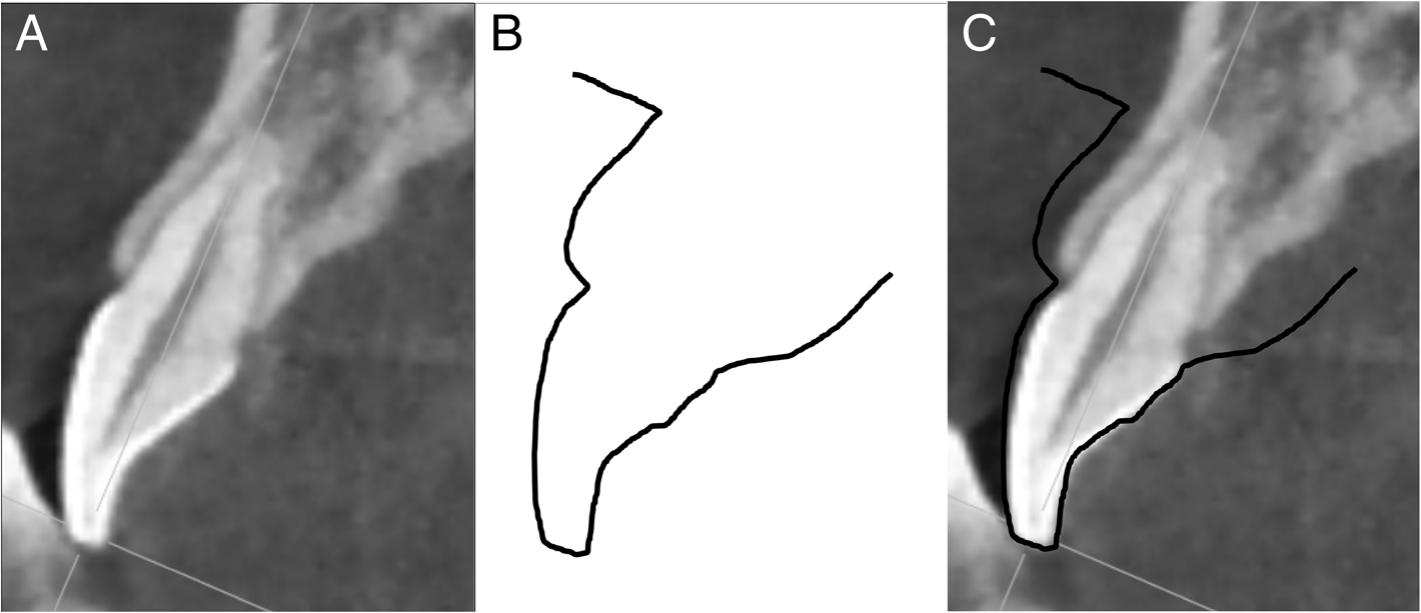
Superimposition of cast and cone beam computed tomography images. a The cone beam computed tomography image. b The scanned cast image. c Superimposition of (a) and (b)
